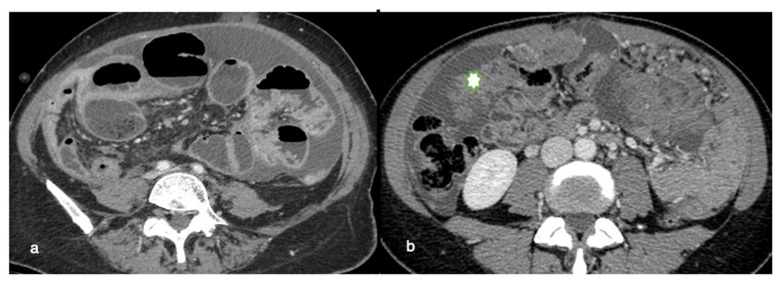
Relative contraindications to PIPAC or laparoscopic approaches. CT axial images can easily detect dilated bowel loops, indicative of intestinal occlusion (a) or diffuse omental thickening (“omental cake”; asterisk) (b). These conditions may represent a relative contraindication to PIPAC, being responsible for complex access to the abdominal cavity with a consequent higher risk for iatrogenic bowel lesions. The presence of omental masses can also potentially reduce treatment efficacy due to difficulties in drug penetration.
